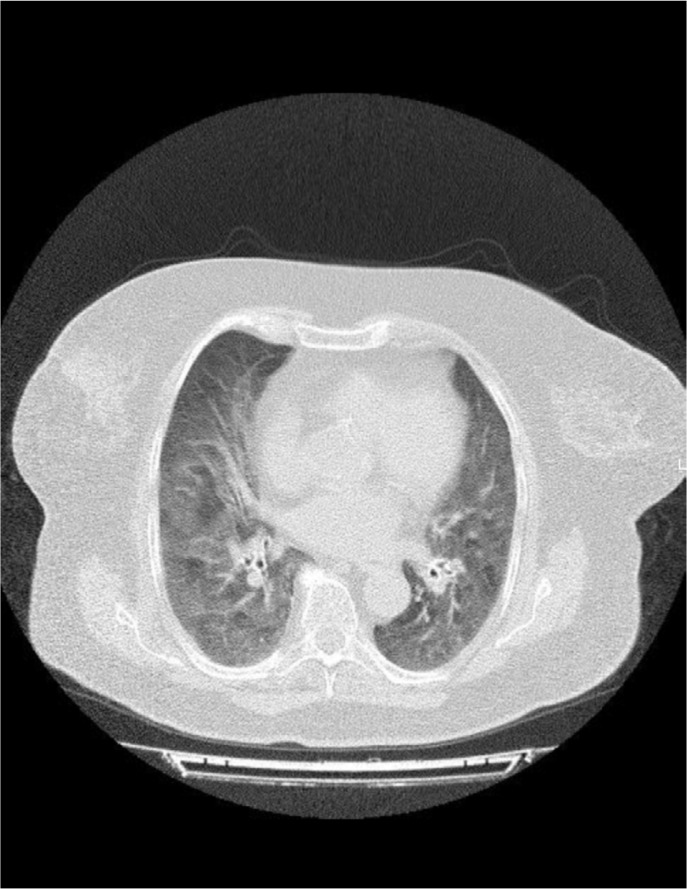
Chest computed tomography at admission showing bilateral ground glass opacities.
